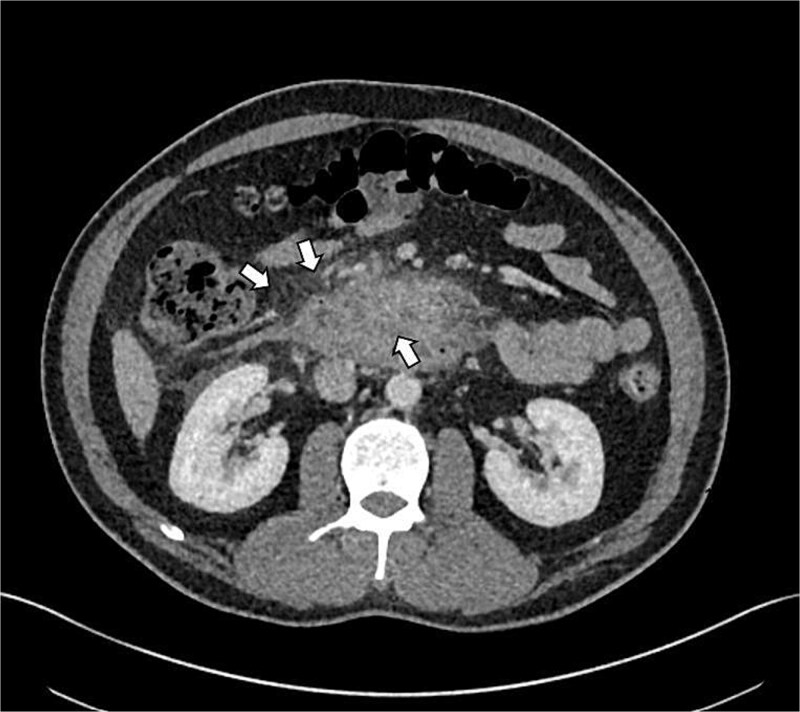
Shows axial CT scan showing prominent reactive mesenteric lymphadenopathy and mucosal oedema in the second and third parts of the duodenum (*indicated by arrows*), consistent with reactive inflammation in GP.
